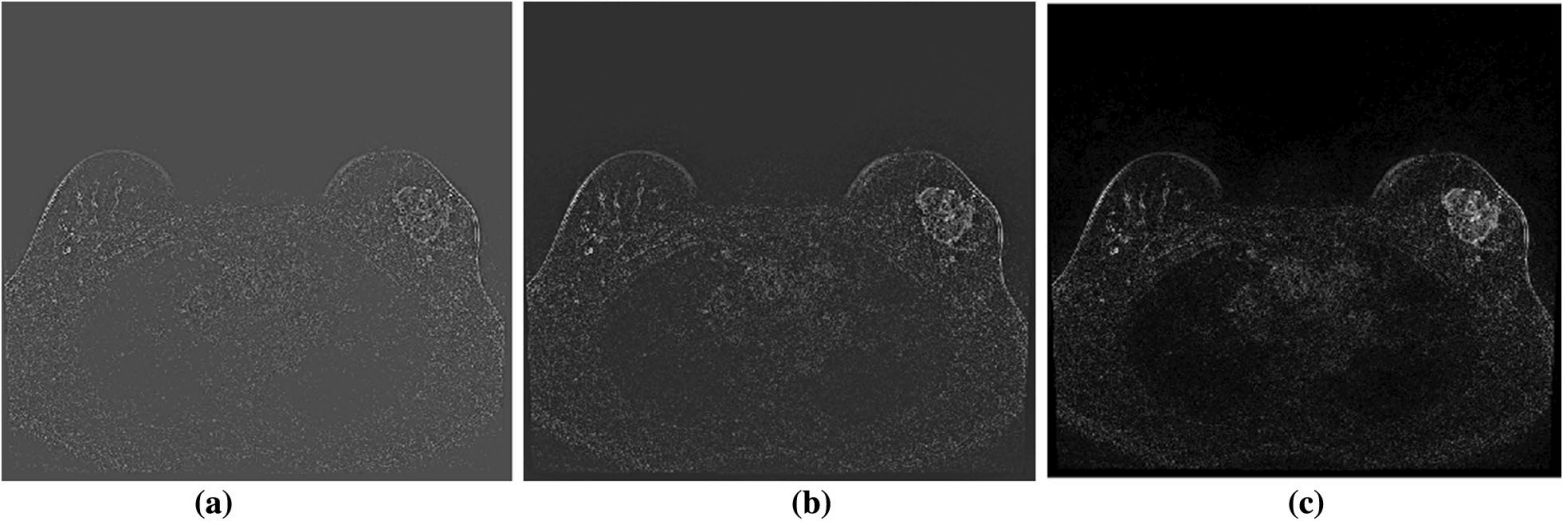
Filter responses of the DCE-MR images obtained according to different scaling factors. a Scaling factor of 1. b Scaling factor of 3. c Scaling factor of 8
